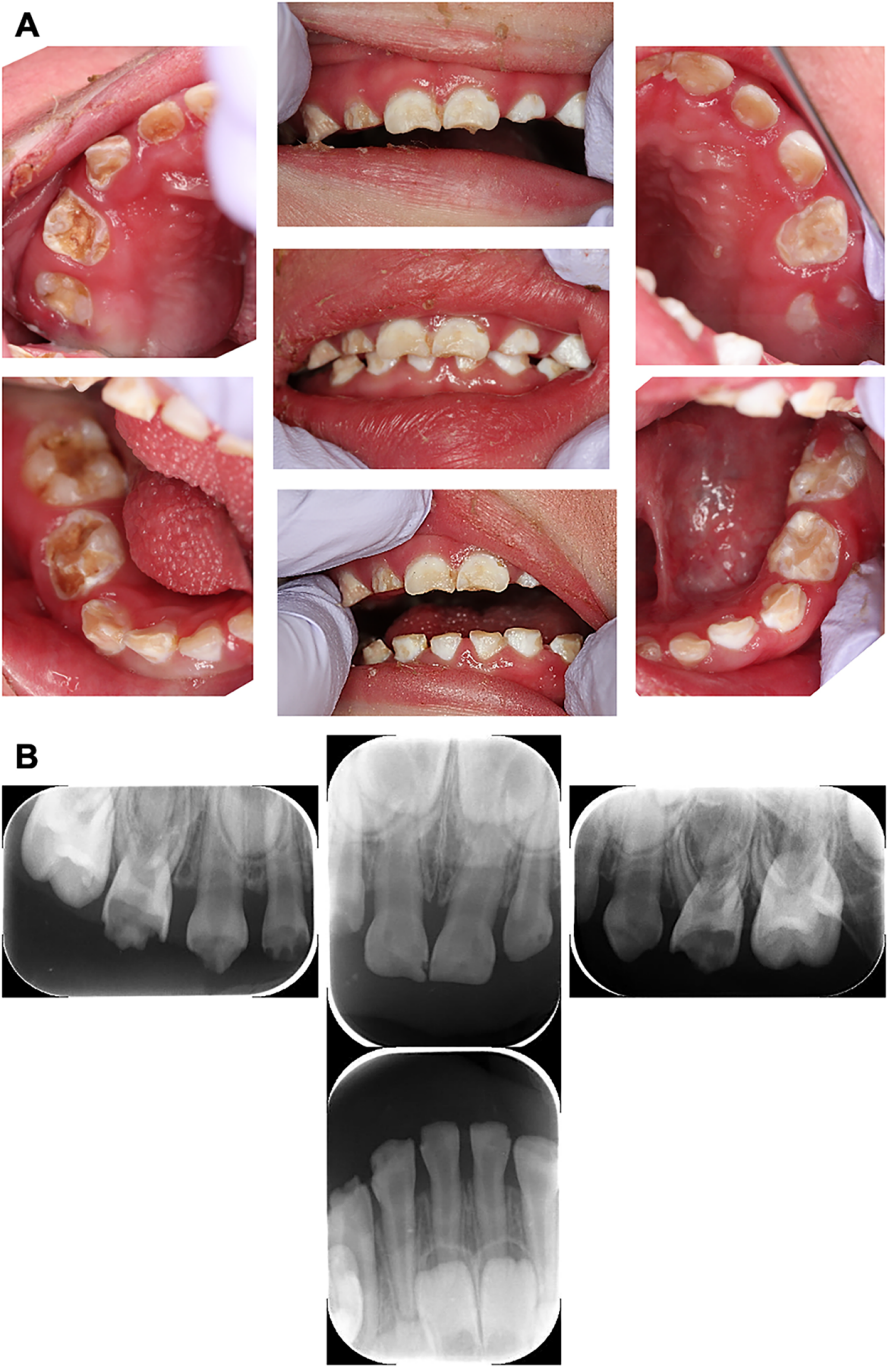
Intraoral and dental x-ray photographs at the initial examination (2 years and 9 months-old). (A) Intraoral photographs at the initial dental examination. Teeth showed numerous opaque white and brown discolourations and defects, diagnosed as multiple severe caries. (B) Pre-treatment dental x-ray image. The mandibular molars were not able to be photographed because the cooperation status of the patient had deteriorated.
